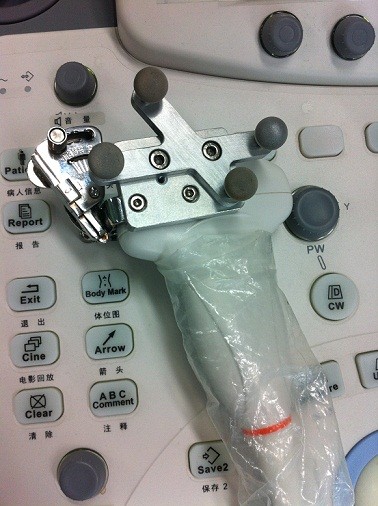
Ultrasound probe mounted with the optically-tracked markers and the puncture trestle.
